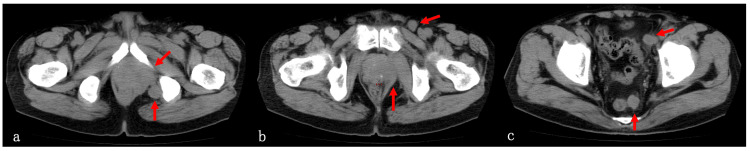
CT image at the initial visit to the Department of Radiation Oncology. (a) Local recurrence, (b) inguinal lymph node metastasis, (c) pelvic lymph node metastasis
